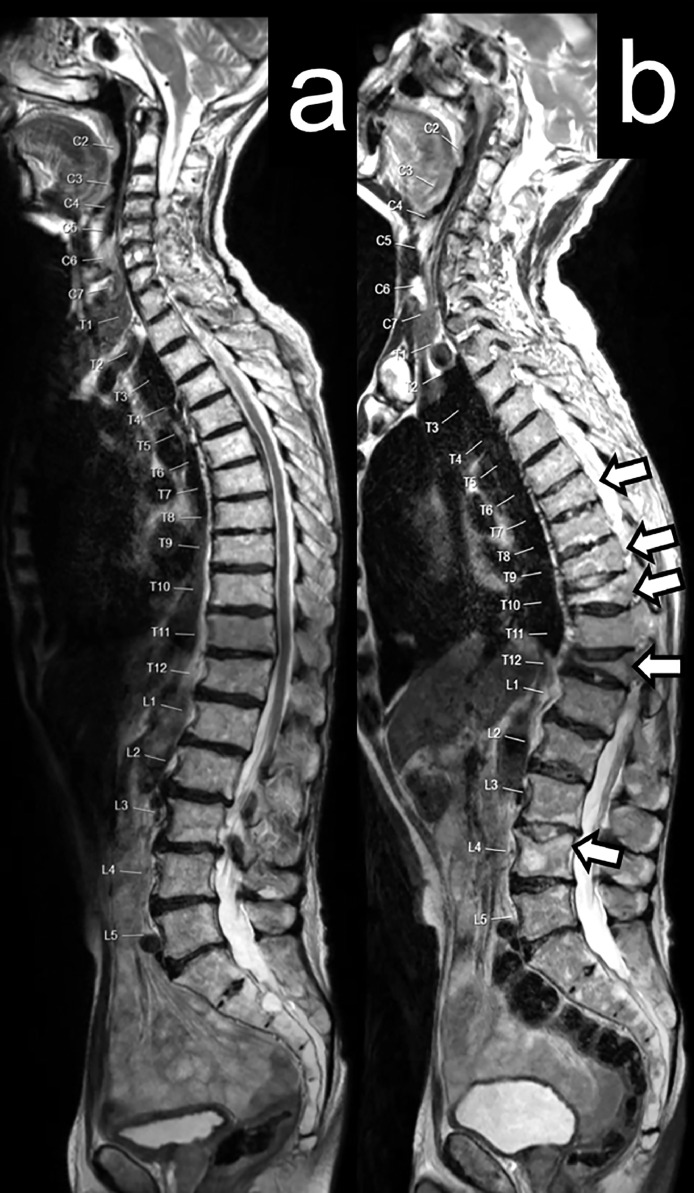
MRI Images Showing New Fractures in a REASURE Patient. Sagittal T2w Composed Spine Images in a 71-Year-Old Man With mCRPC at Baseline (a) and 2 Years After Six Cycles of Radium-223 (b). Follow-Up Images Show Multiple Non-Malignant Endplate Vertebral Fractures at T7, T8, T9, T10, T12, and L4 (White Arrows) With Significant Vertebral Collapse at T10 and T12. Abbreviation: MRI = magnetic resonance imaging; mCRPC = metastatic castration-resistant prostate cancer.
